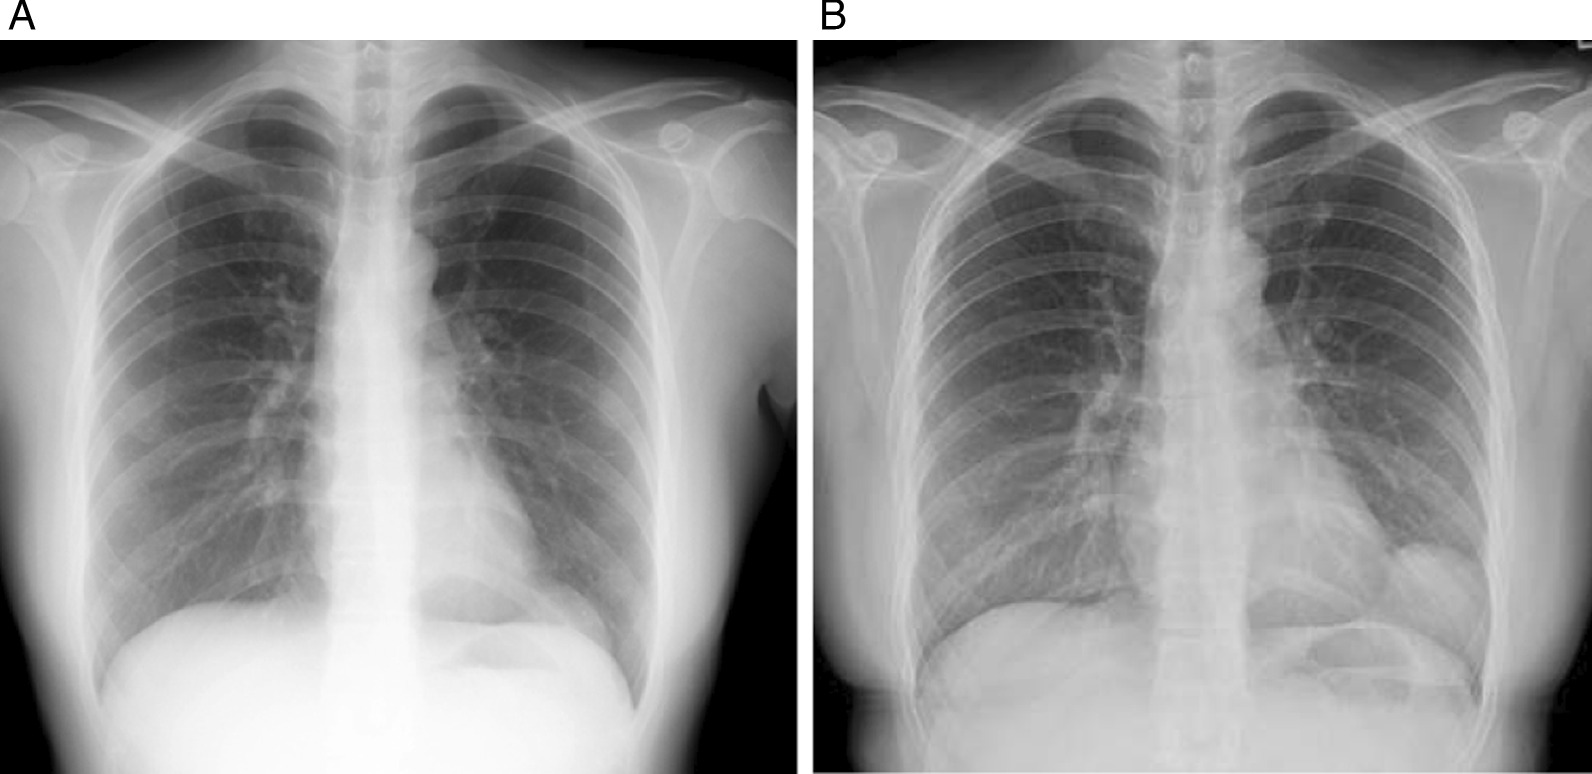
Chest X-ray taken in A 2018 and B 2019. The tumor size enlarged from 34 to 64 mm in the largest diameter
